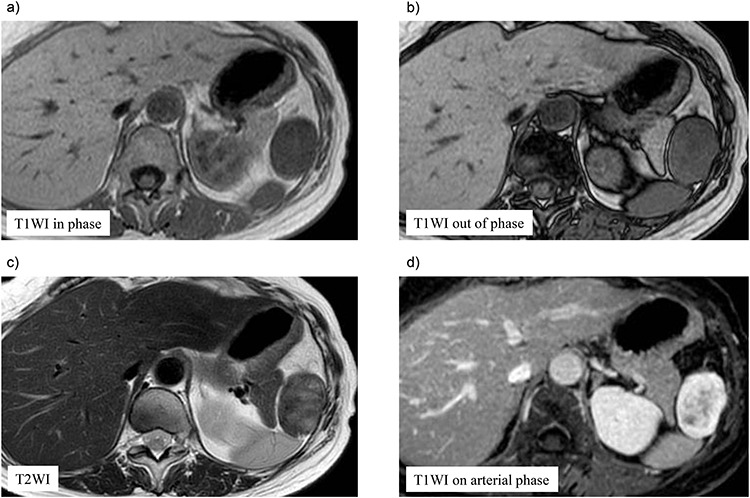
Preoperative magnetic resonance (MR) imaging findings. The tumor shows low signal intense mass on T1-weighted MR images in phase (a) and out of phase (b) and a heterogeneous hyperintense mass on T2-weighted MR images (c). (d) The tumor shows hypointense tumor on T1-weighted MR images with delayed enhancement in the arterial phase.
